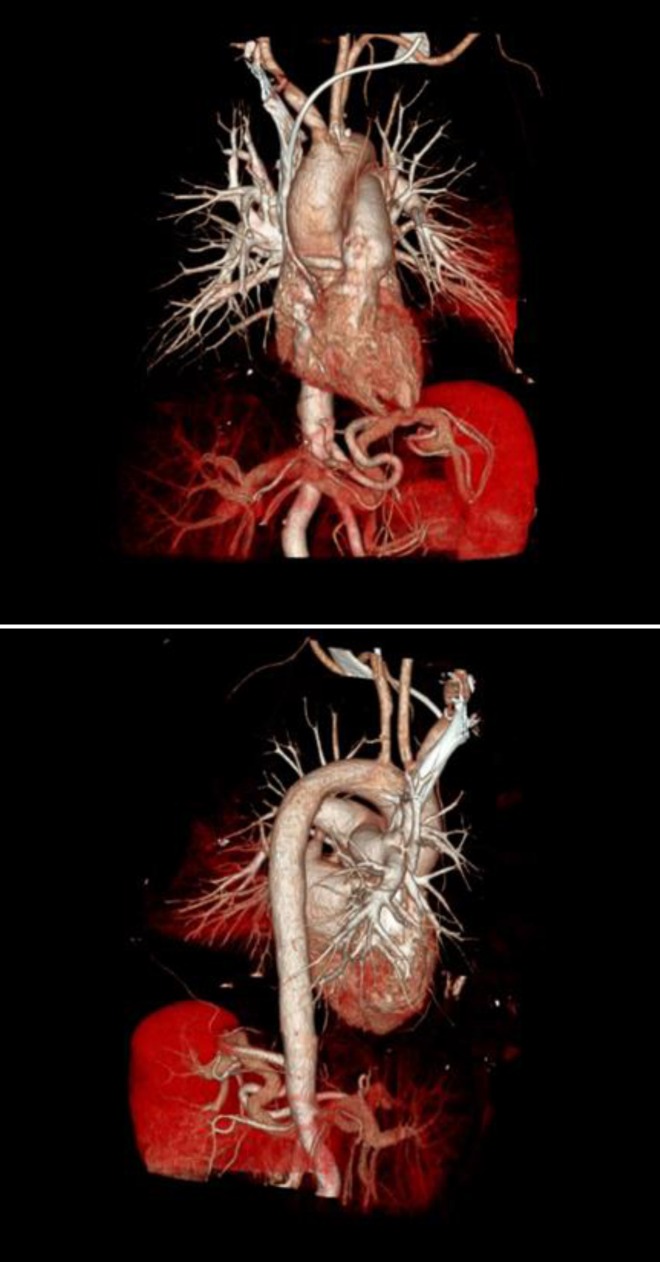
CT angiography of arterioesophageal fistula on the left subclavian artery
